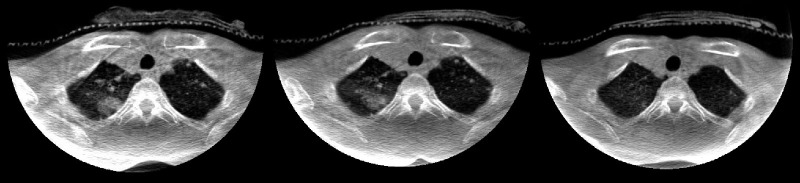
Cone beam computed tomography (CBCT) images CBCT used for patient radiation treatment positioning purposes (not diagnostic). The images are obtained from CBCT scans prior to every treatment. From left to right, images were taken on October 19th, 26th, and November 9th, 2016 respectively.
